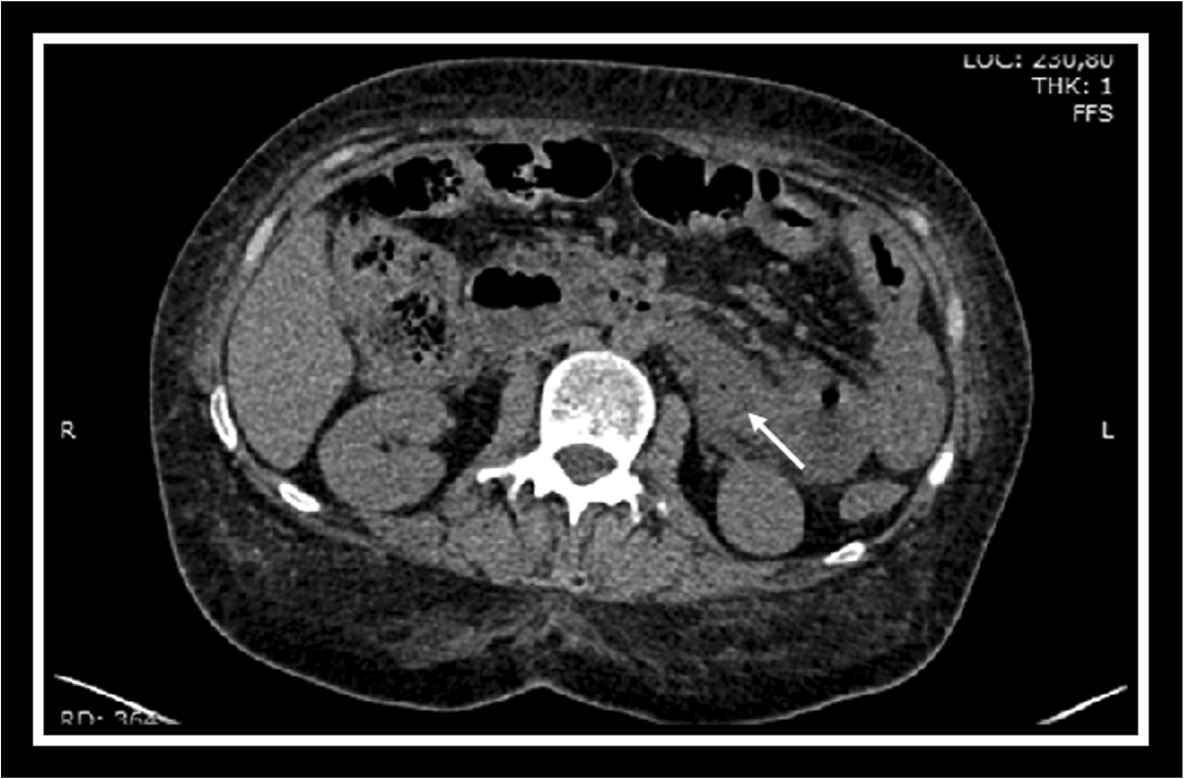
Abdominal computed tomography (CT) scan showed free retroperitoneal air (arrow), suspected for a small leakage from the posterior aspect of the third duodenal portion.
